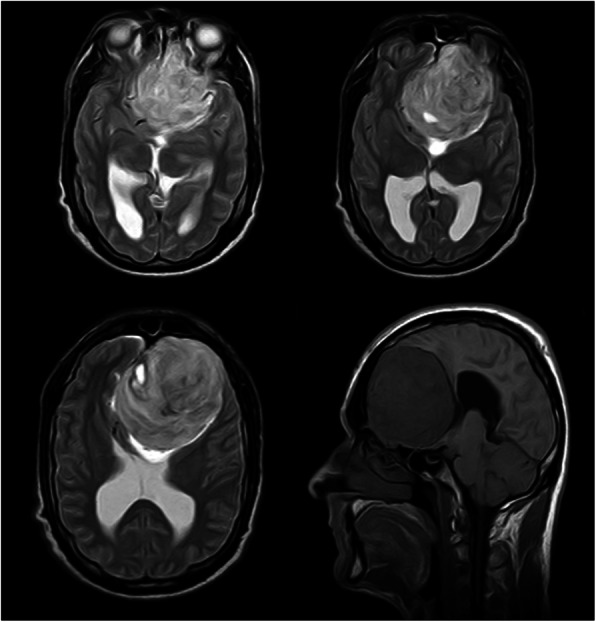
a, b, c Axial T2 brain MRI images showing a predominantly left frontal tumor lesion, with compression of the optic chiasma; d) Sagittal T1 brain MRI image showing frontal tumor lesion and compression of the anterior skull base structures
